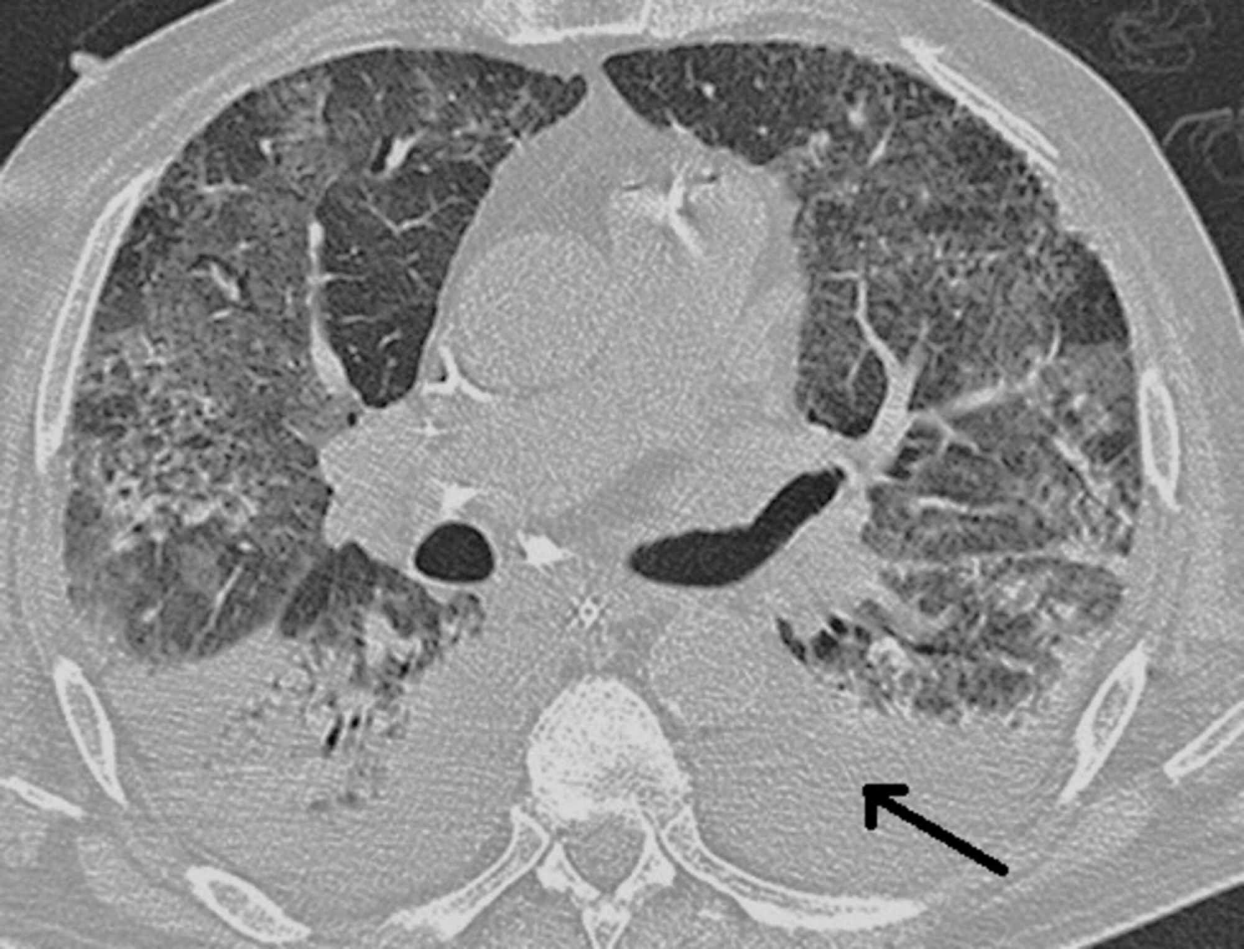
Chest CT scan without IV contrast of the patient with coronavirus infection establishing severe ARDS. CT: Computer Tomography; IV: Intravenous; ARDS: Acute Respiratory Distress Syndrome.
